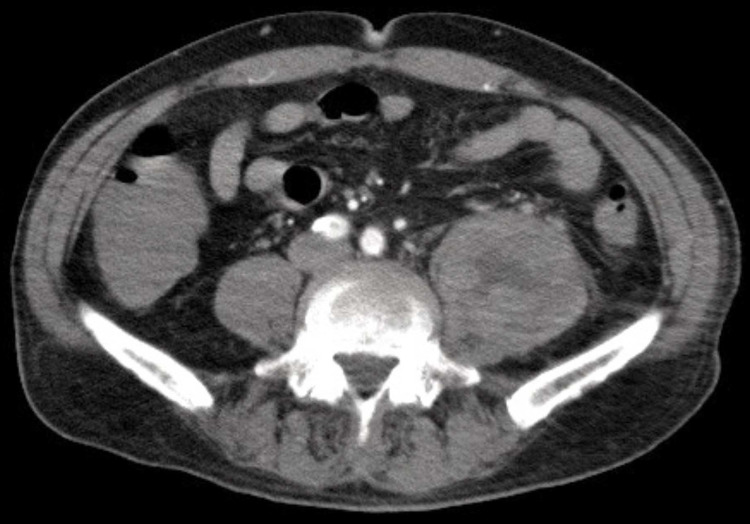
Computed tomography on day 26 Follow-up enhanced computed tomography on day 26 revealed no apparent progression of left iliopsoas hematoma, and no extravasation of contrast media was seen.
